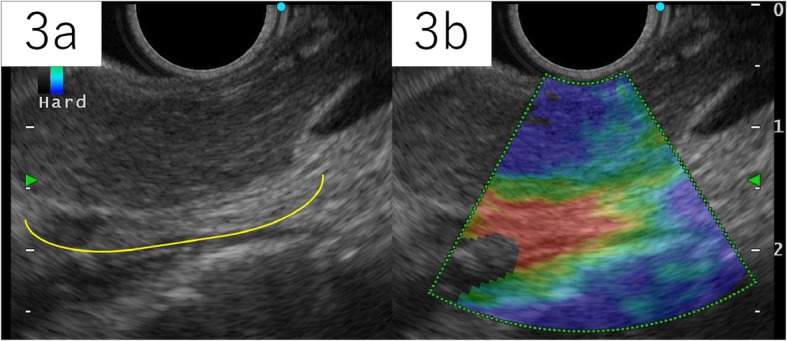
Endoscopic ultrasonography (EUS) depicted well-demarcated mass (indicated with arched line) with inside mosaic pattern (3a), and the mass was described harder than the parenchyma of the pancreas by elastography (3b)
